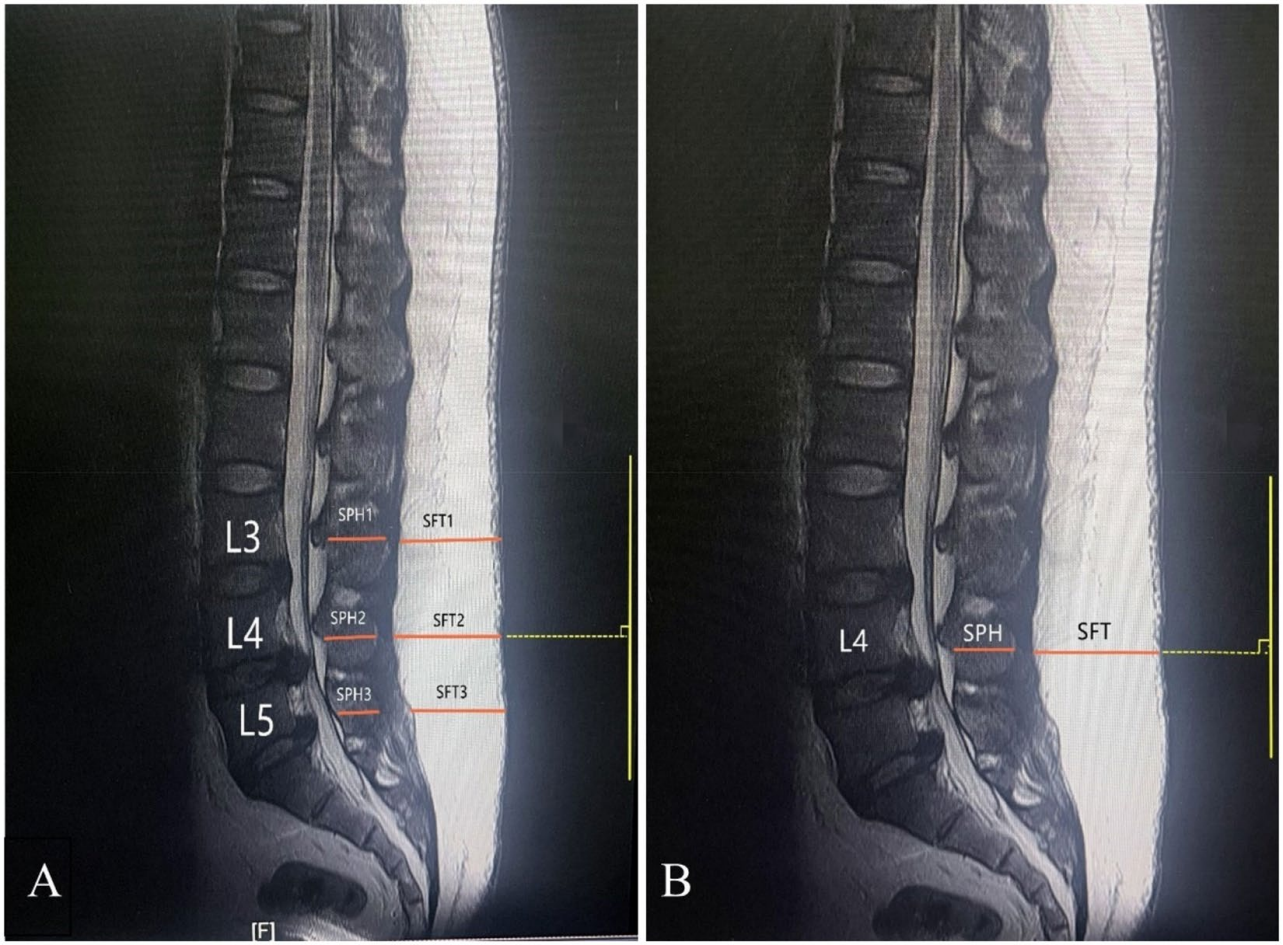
The measurements of lumbar subcutaneous fat thickness (SFT) and spinous process height (SPH) at the operated levels (excluding S1) on T2 midsagittal MRI. A: Traditional measurement: SLSI=(SFT1/SPH1 + SFT2/SPH2 + SFT3/SPH3)/3. B: New predictor: MSLSI: The measurements only at the level of L4.
